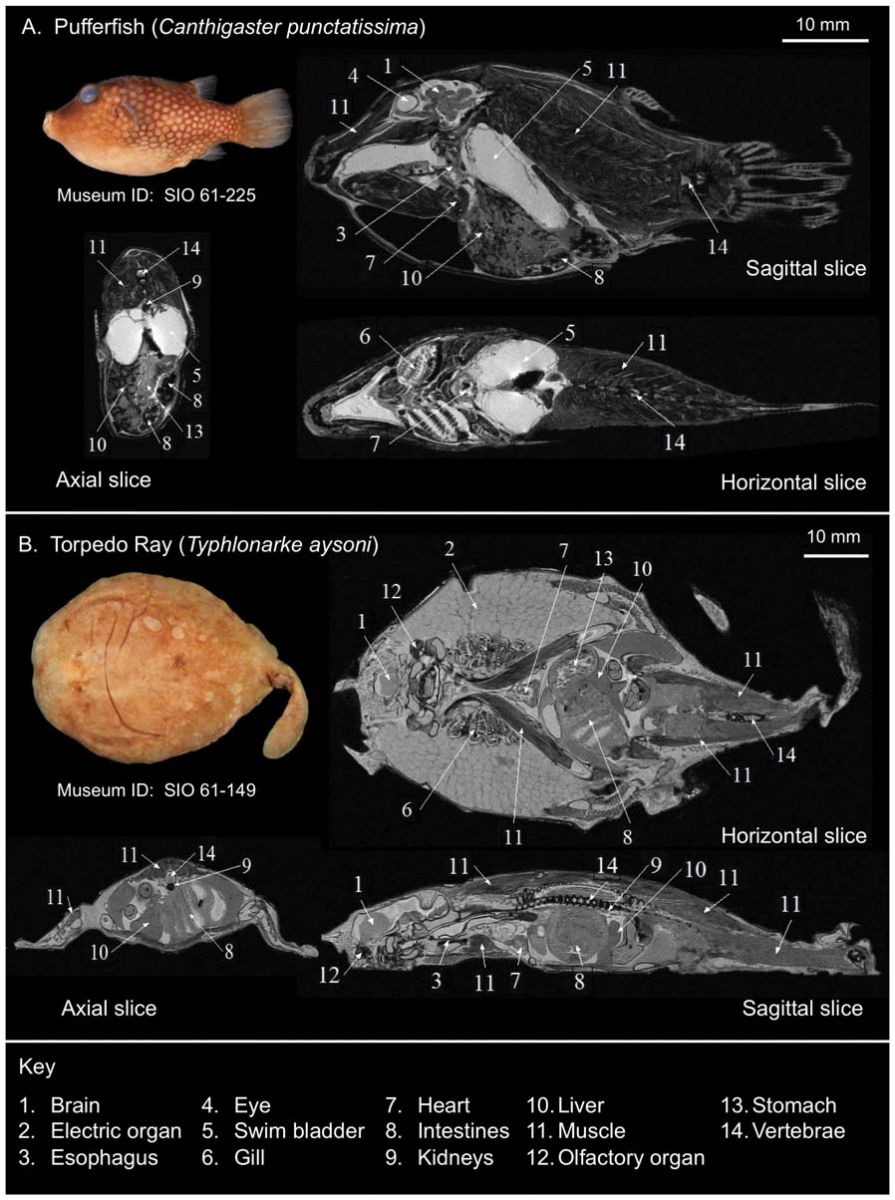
Examples of T1-weighted contrast-enhanced 7T MRI data. (A) Spotted sharpnose puffer, *Canthigaster punctatissima* (SIO 61-225, SL 57 mm). Pulse sequence parameters include: FA = 15°, TR = 22.814 ms, TE = 11.322 ms, slice thickness = 100 µm, slice matrix = 354×780×210, resolution = 100 µm^3^, averages = 5. (B) Blind legged torpedo, *Typhlonarke aysoni* (SIO 61-149, SL 92 mm). Pulse sequence parameters include: FA = 15°, TR = 23 ms, TE = 11.222 ms, slice thickness = 100 µm, slice matrix = 550×830×180, resolution = 100 µm^3^, averages = 3. Numbered labels indicate a selection of readily visible anatomical structures in these slices.
